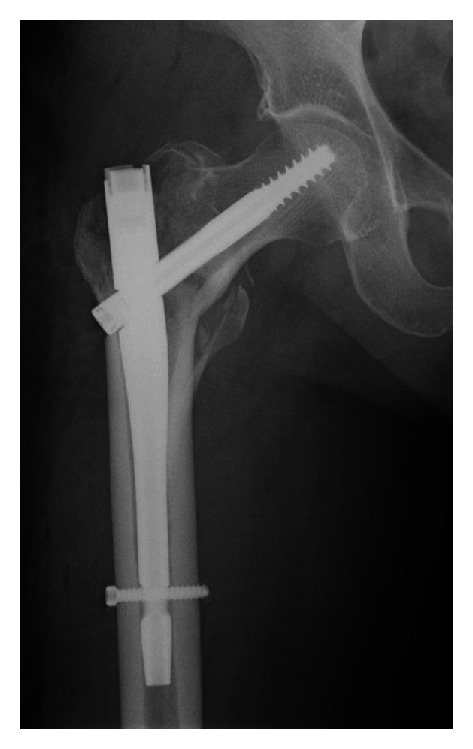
Radiograph showing insufficient reduction of the trochanteric fracture after implantation of the Gamma 3 nail.
